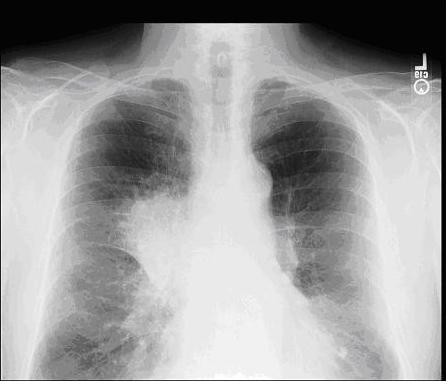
PA chest radiograph reveals a right hilar mass.
